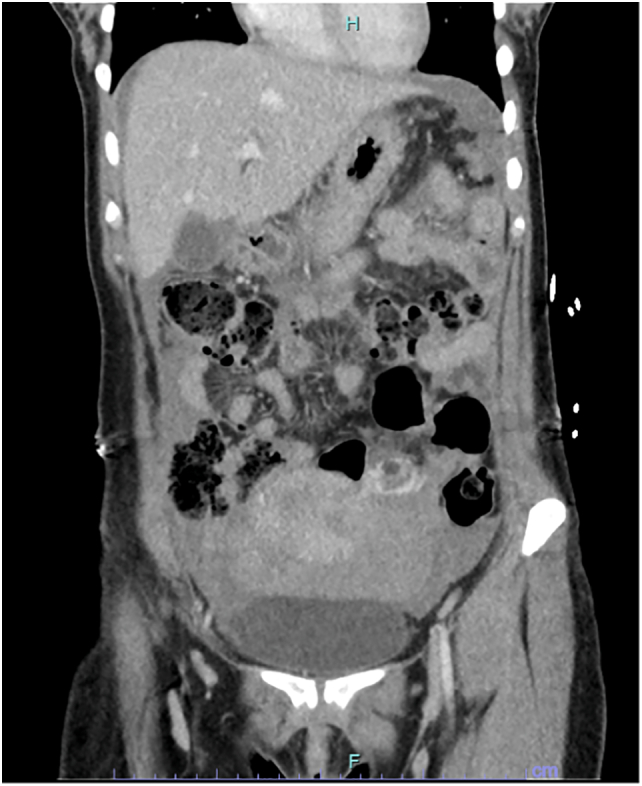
CT scan of the abdomen showing free fluid in the pelvis and upper abdomen.
